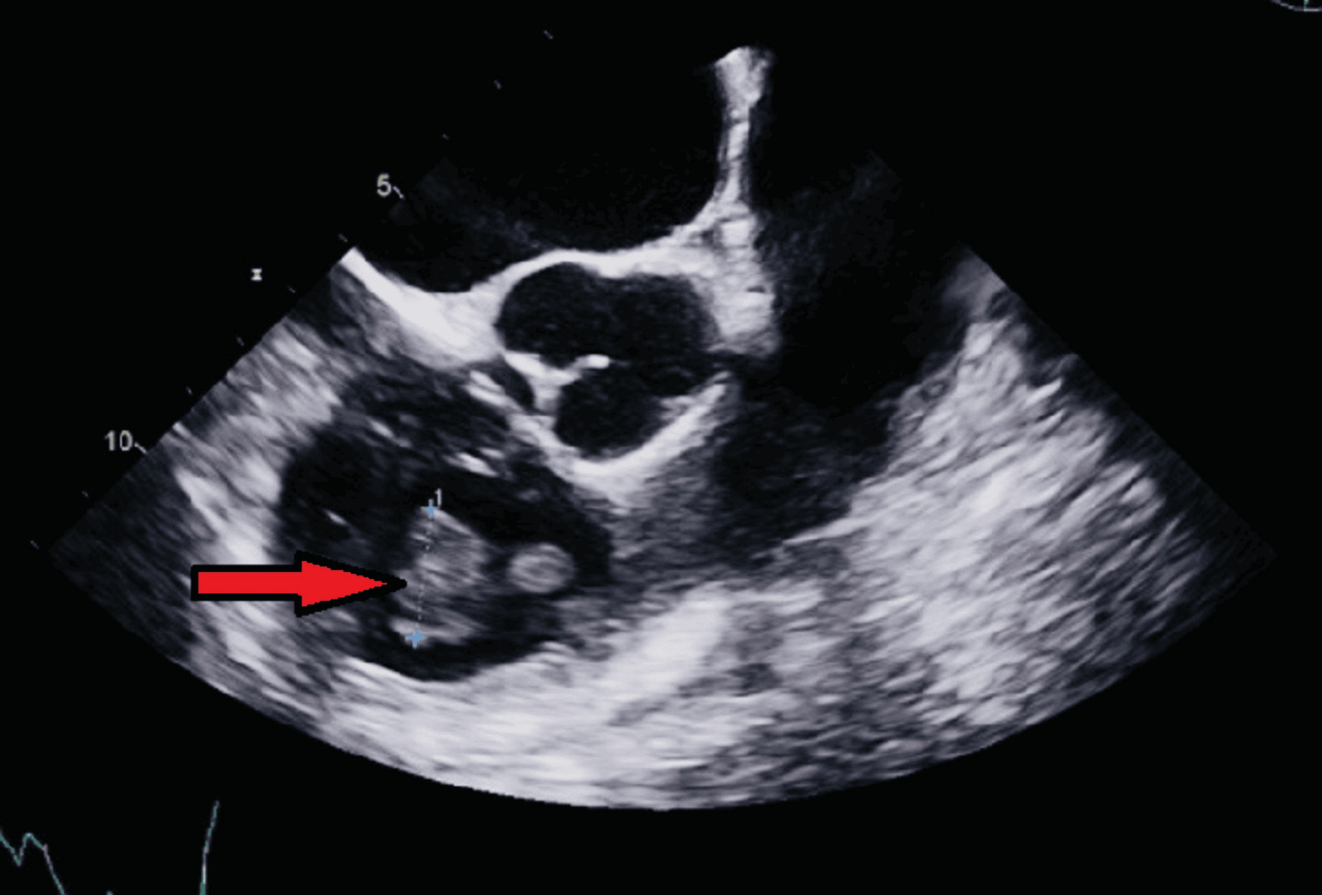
Transthoracic echocardiogram revealed a tricuspid valve vegetation extending into the proximal right ventricular outflow tract measuring 1.9 cm.
